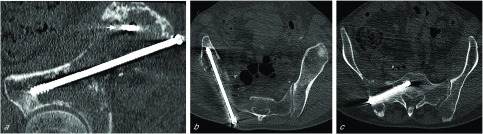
(a) Oblique sagittal reformatted view of the pelvis CT scan (bone window, slice thickness 1 mm) acquired after osteosynthesis (first step of the procedure). The first screw was placed into the iliac bone from the posterior iliac crest to the anterior inferior iliac spine along the arcuate line. The cross section of the proximal part of the second screw inserted into the sacroiliac joint is visible (small arrow). (b) Oblique axial reformatted view of the pelvis CT scan after iliac screw fixation. (c) Oblique axial reformatted view of the pelvis CT scan after sacral screw fixation. This second screw was inserted into the sacroiliac joint to the sacral wing.
